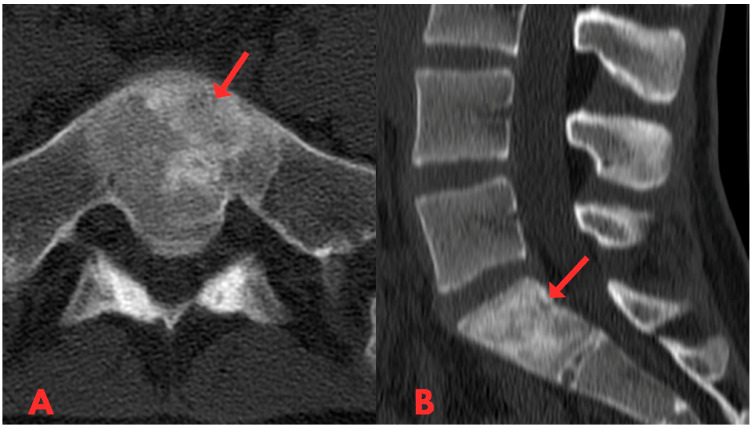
First CT examination of the lumbar spine axial (A) and sagittal (B) planes, showing heterogeneous sclerotic changes in the body of the S1 vertebra (red arrow).
